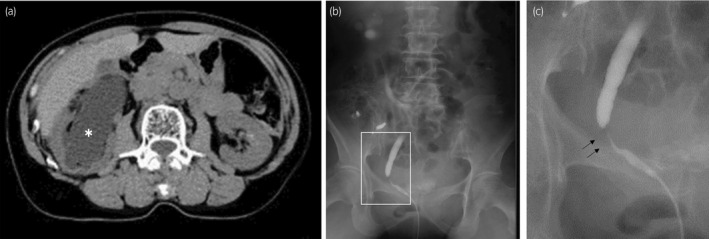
Right renal hydronephrosis and atrophic kidney detected by CT examination (a; asterisk). Retrograde pyelography shows a 15‐mm distal ureteral stricture (b and c; arrowhead).
